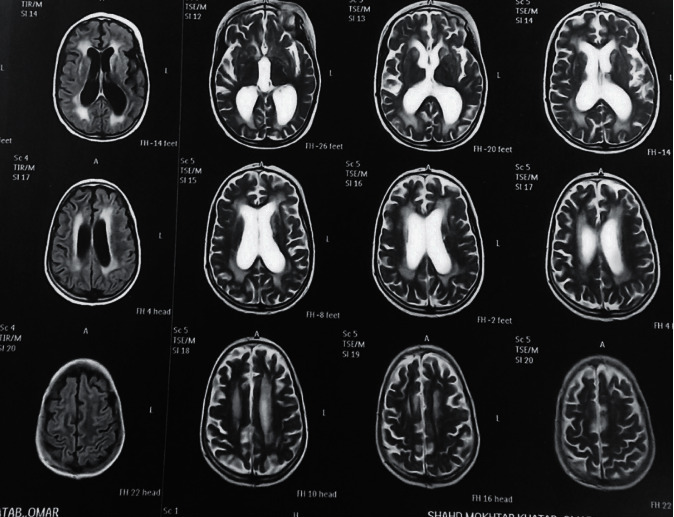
MRI brain performed at the time of 3rd admission; dilated third ventricles were evident due to brain cell atrophy; at this occasion, signs of increase intracranial tension were negative which excluded it as a cause for cerebral ventricles dilatations.
